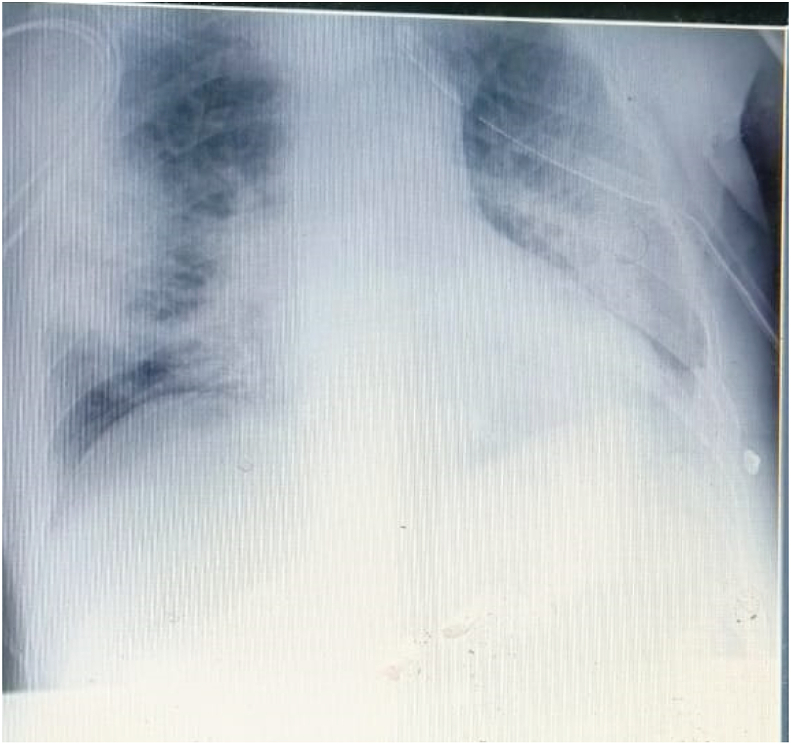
Chest -X-Ray realized after pneumothorax drainage.
